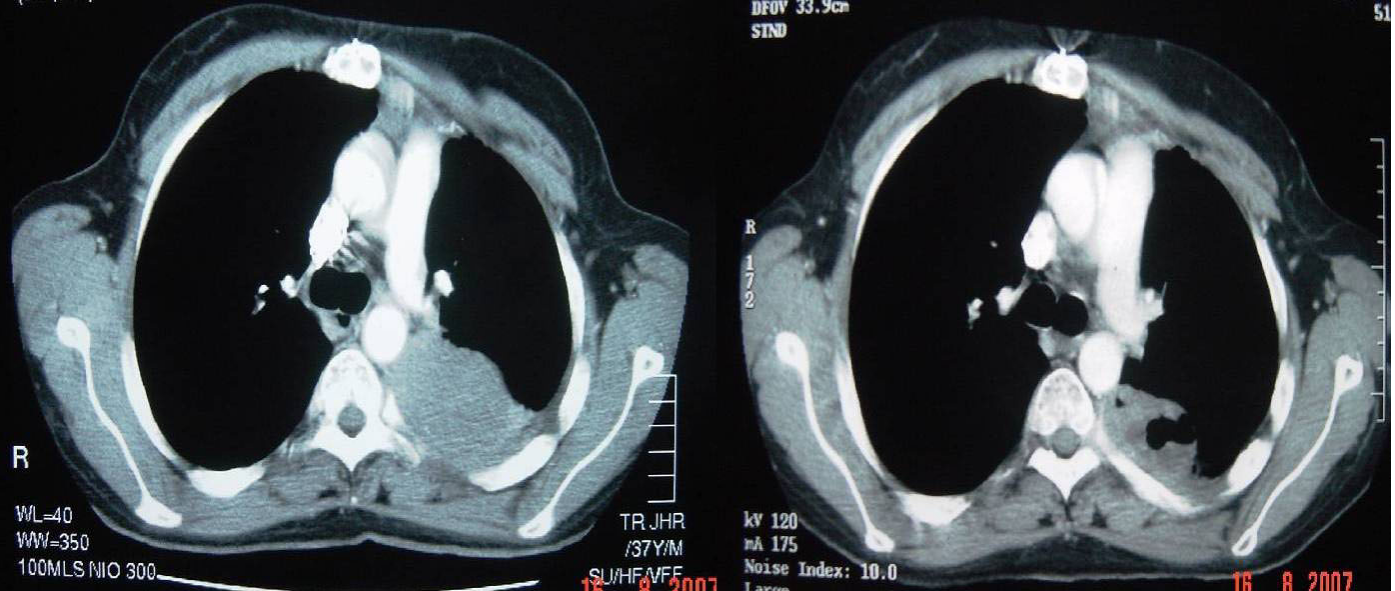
Pre- and post-treatment CT scans at the level of the carina.
